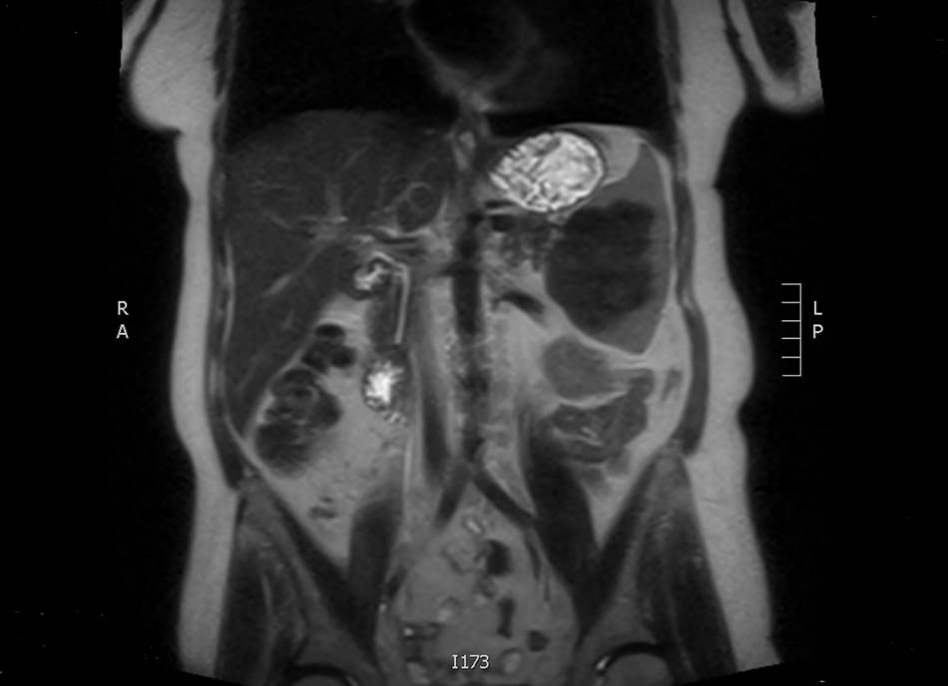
MRI image of a splenic mass
